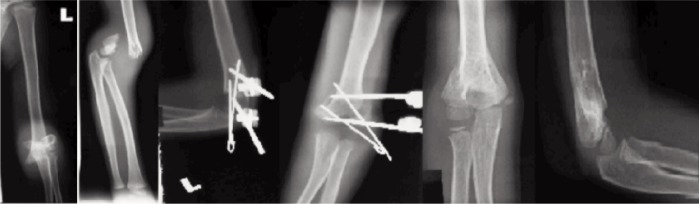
Serial radiographs of a left humeral supracondylar fracture Gartland type III successfully treated with lateral external fixation and Kirschner wiring.
